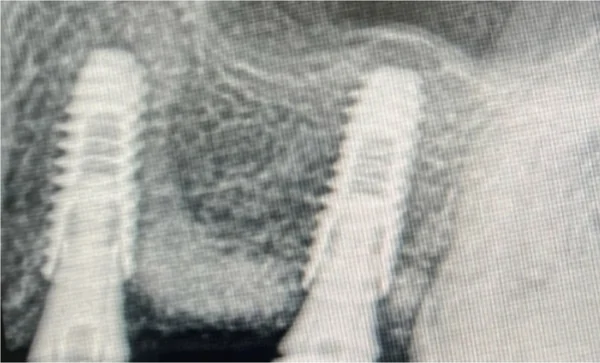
Radiograph showing successful osseointegration of the implant after the healing period, with healing abutments in place.
